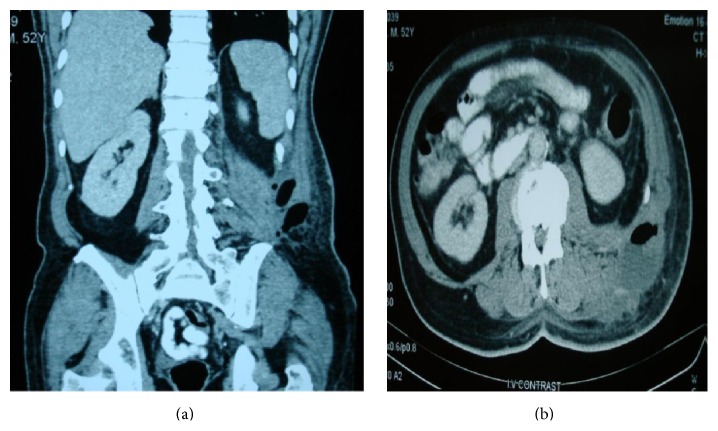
Abdominal CT scans revealed a perforated diverticulitis of the sigmoid colon causing subcutaneous inflammation and free gas in the tissue of the posterior abdominal wall and lumbar area.
